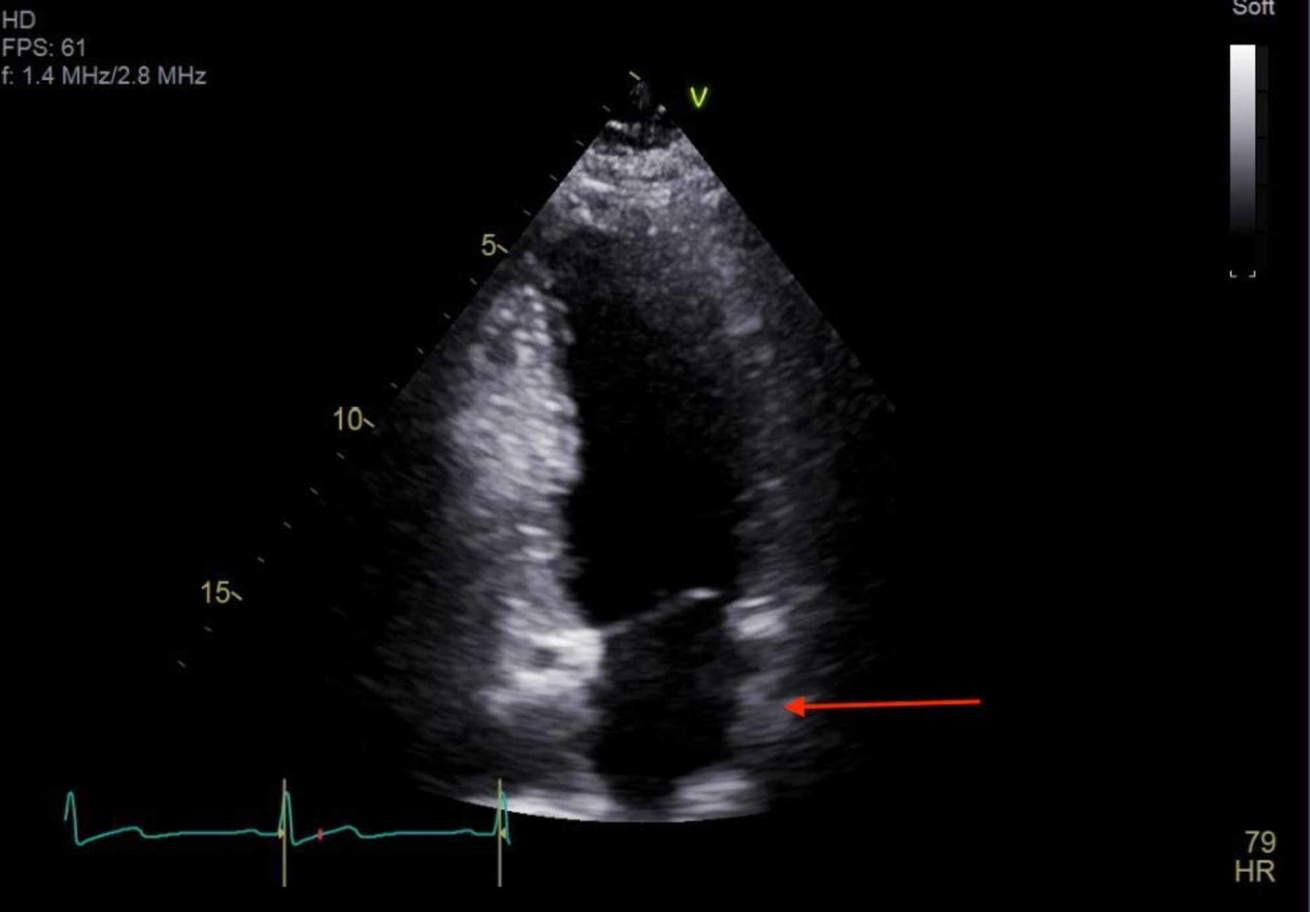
Transthoracic echocardiogram repeated one month later showed thickening (red arrow) near the left atrial wall due to known remnants of the bronchogenic cyst.
